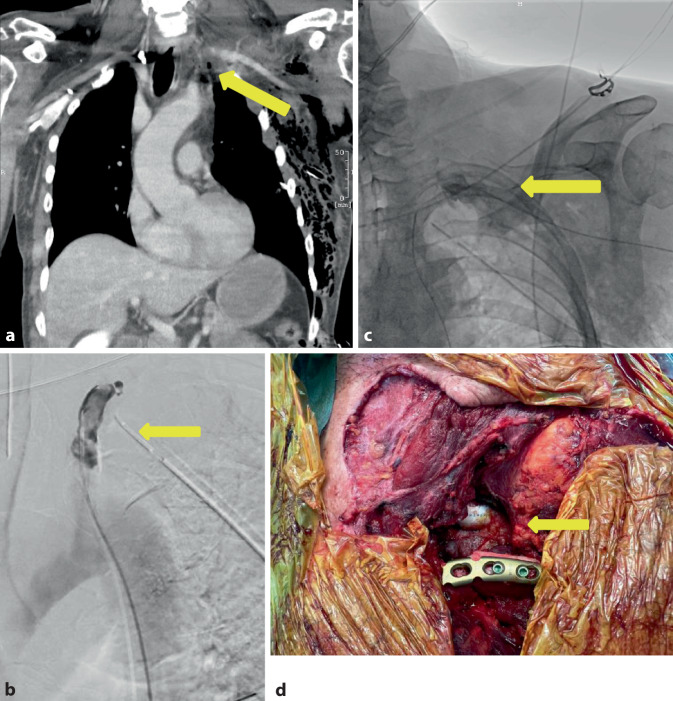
Fall 4: Traumatische Verletzung der A. subclavia links. a CT-Angiographie mit Nachweis des Verschlusses der A. subclavia links (*gelber Pfeil*), b Angiographie mit Versuch der Sondierung des Verschlusses vom Abgang aus der Aorta (*gelber Pfeil*), c Angiographie mit Sondierung des Verschlusses im Rendesvouz-Verfahren zusätzlich von transbrachial (*gelber Pfeil*), d Operationssitus mit sichtbarer PTFE-Prothese und rekonstruierter Klavikula mit Platte (*gelber Pfeil*)
